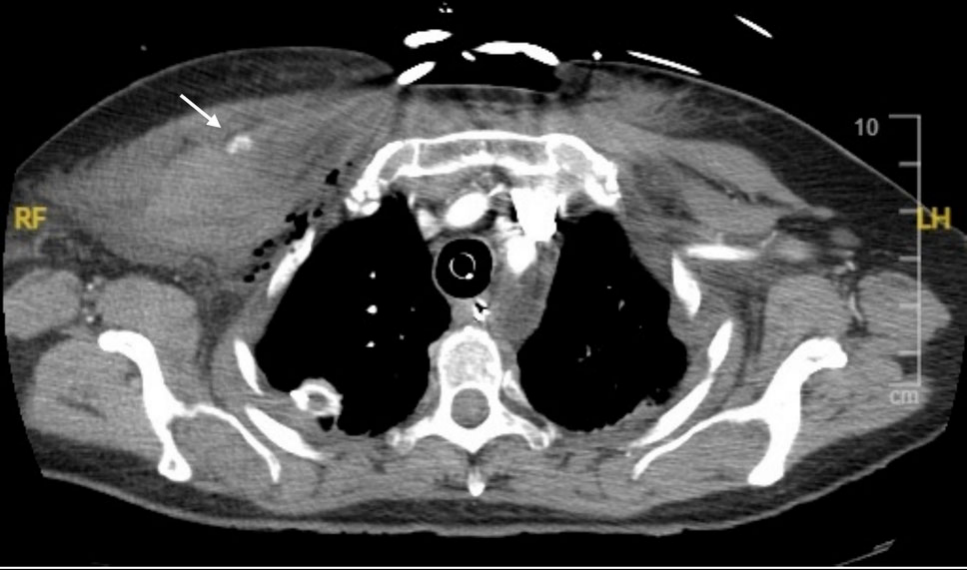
44-year-old male presenting to the emergency department after a motor vehicle accident. Amongst multiple other injuries, the CT scan demonstrated a right pectoral intramuscular haematoma with contrast extravasation (arrow). The patient was treated with endovascular embolisation
